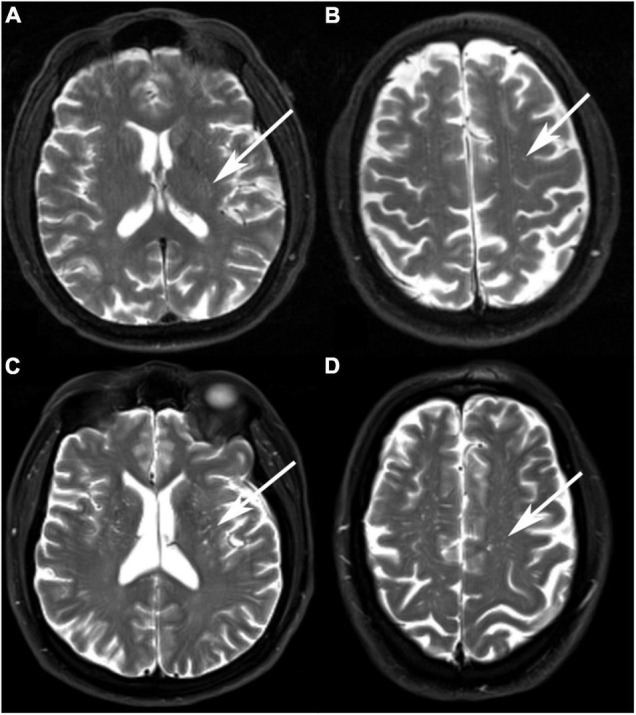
Examples of MRI-visible perivascular spaces. (A,B) A 68-year-old Vietnam war veteran without a history of traumatic brain injury (TBI) had a low degree of BG-PVS and CSO-PVS. (C,D) A 67-year-old Vietnam War veteran with a history of TBI had a high degree of BG-PVS and CSO-PVS.
